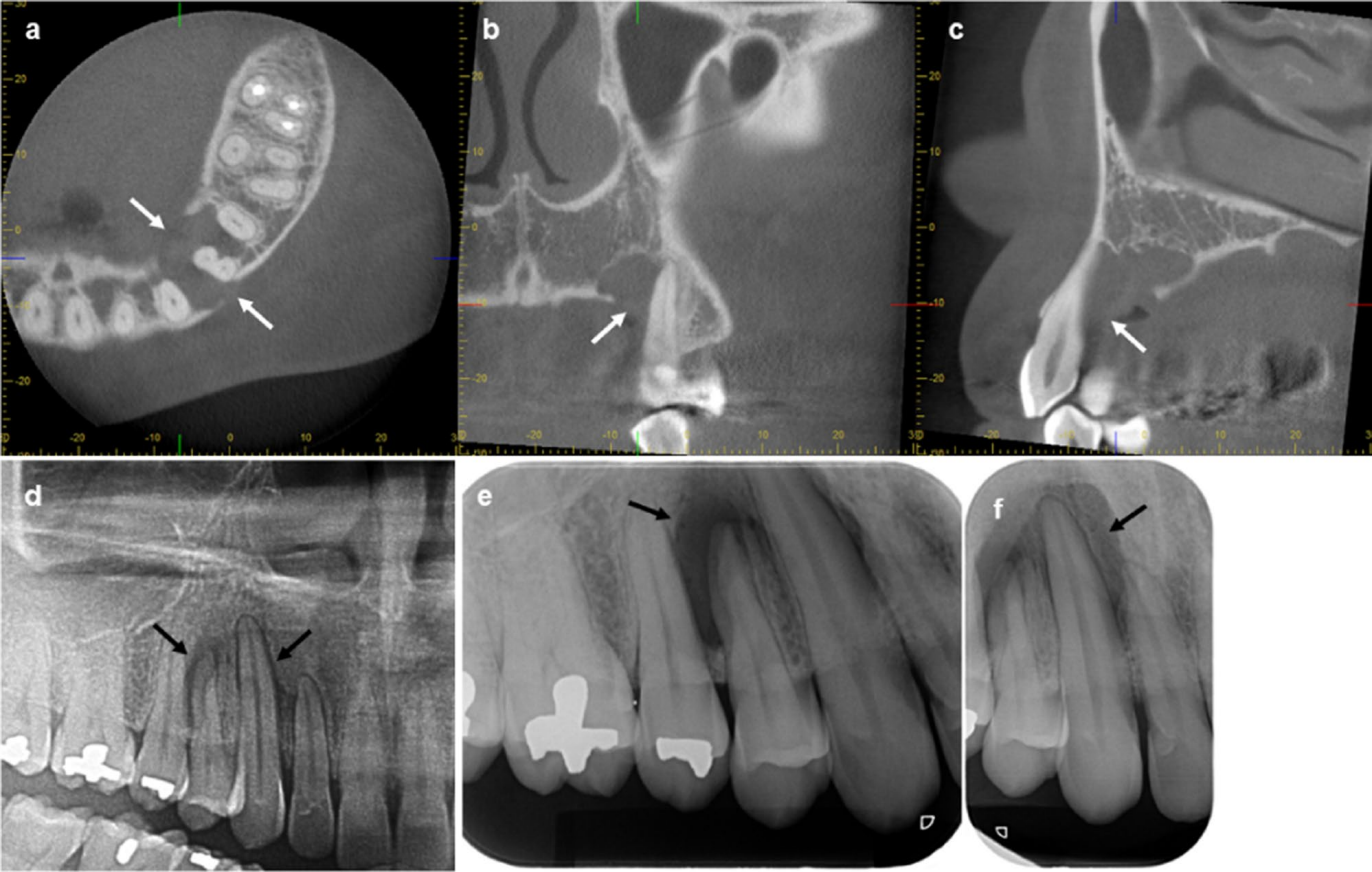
Radiographs of case 1 (a-c) and case 2 (d-f). Cone beam computed tomography images in axial view (a), coronal view (b) and sagittal view (c) show a maxillary lesion with resorption of the bone around teeth without tooth displacement or resorption. Perforation of buccal and palatal cortices (white arrows) is demonstrated without bone expansion. A cropped panoramic image (d) and periapical images (e and f) show a periapical radiolucent lesion extending from distal surface of the upper right lateral incisor to the mesial surface of the upper right second premolar (black arrows) mimicking periapical inflammatory lesion
